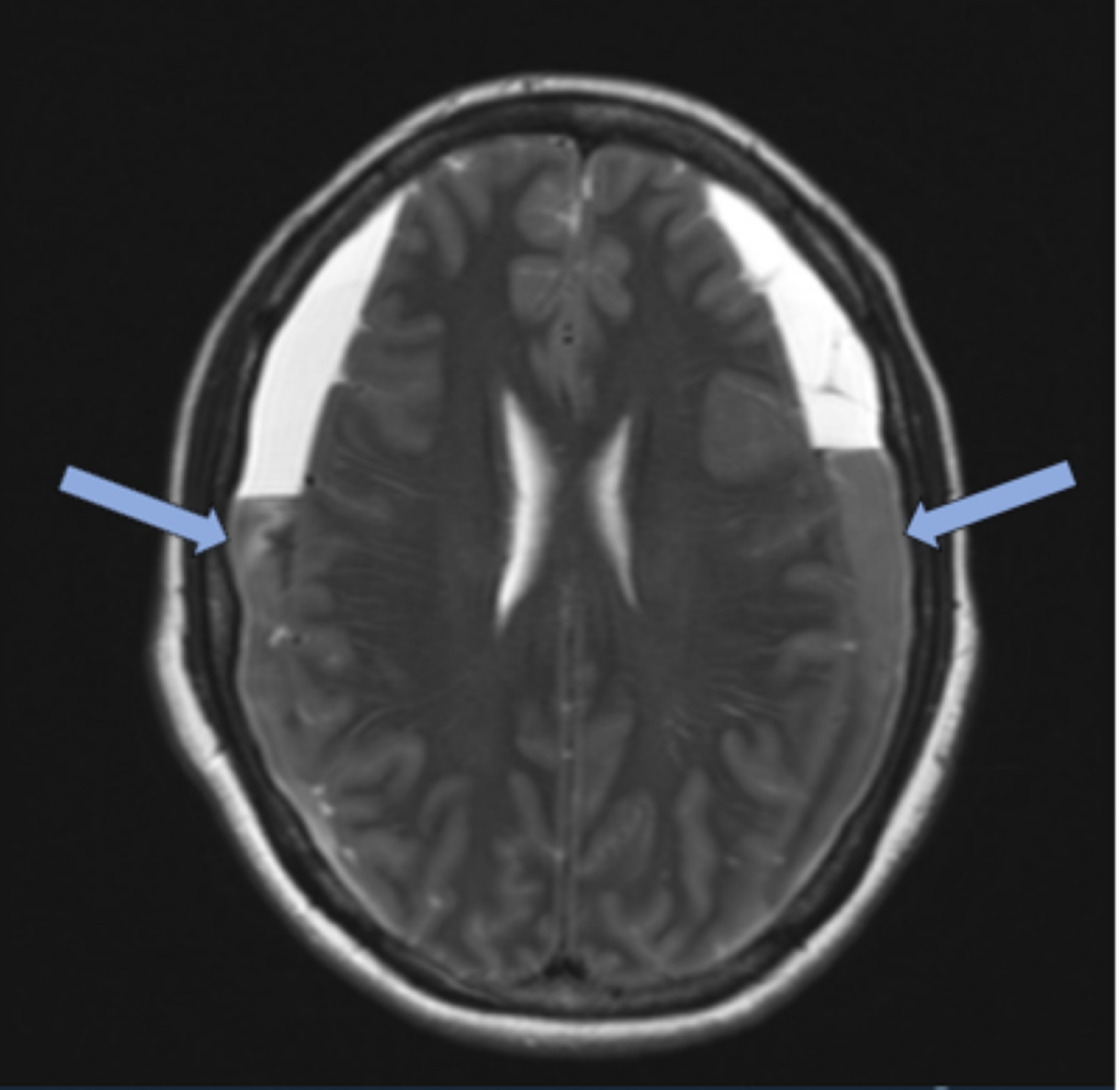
Axial slice of T2 magnetic resonance imaging demonstrating bilateral subacute subdural hematomas (blue arrows) with confirmed intracranial hypotension from cerebral spinal fluid leak.
